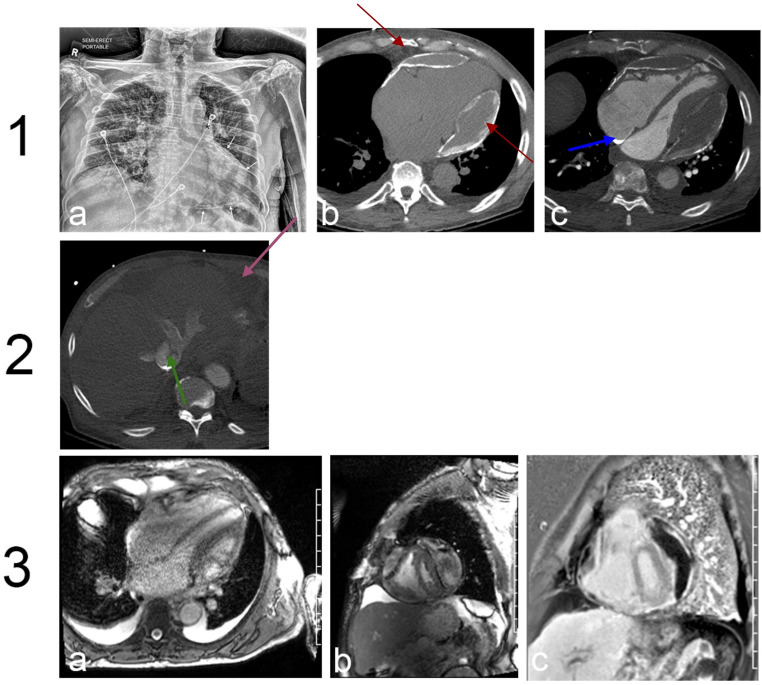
[1]: Chest radiograph depicting cardiomegaly (white arrows) (a). CT angiography in axial view showing dense calcification involving both the visceral and parietal pericardial layers (red arrows) (b). A moderate-sized, loculated pericardial effusion with internal calcific density is seen (blue arrow) (c) [2]: CT angiography in axial view depicting reflux of contrast (green arrows) into the inferior vena cava. Also depicted is hepatomegaly with heterogenous “nutmeg” appearance of the liver parenchyma and predominantly simple-fluid density ascites (pink arrows) (a, b) [3]: Cinematic views of cardiac MRI showing compression of the LV cavity by a thickened and fluid filled pericardial sac and septal bounce (a, b). DGE sequence demonstrates pericardial enhancement (c)
